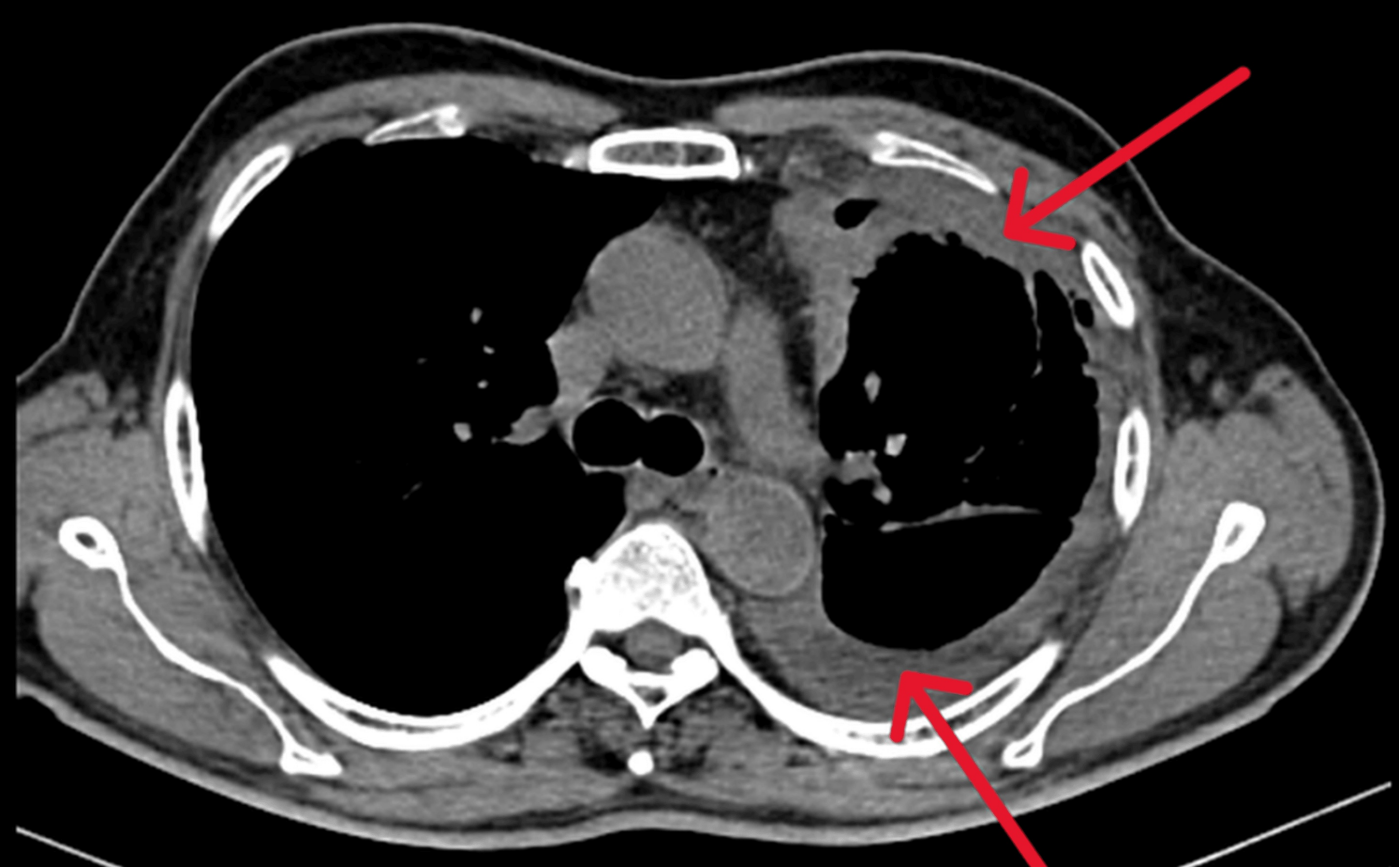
CT scan of thorax suggestive of pleural thickening (red arrows) CT: computed tomography
